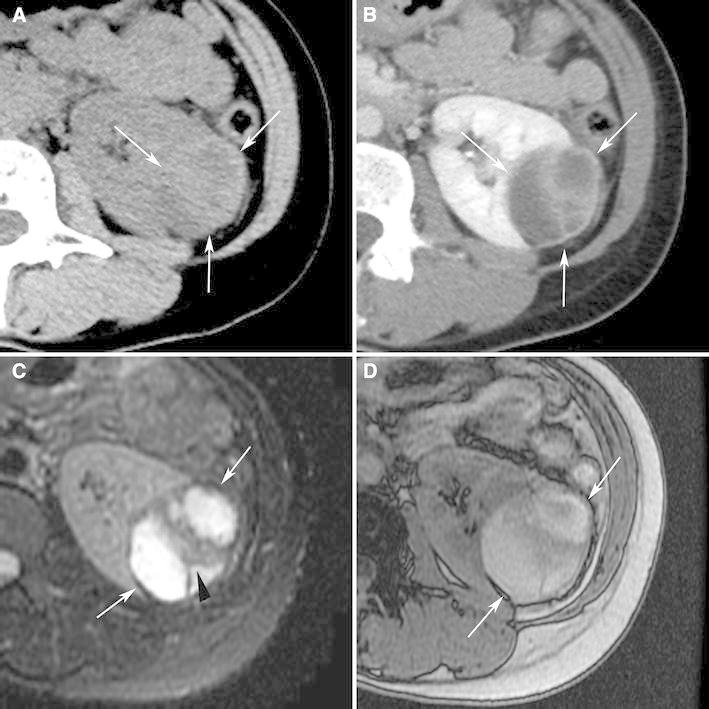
Epithelioid angiomyolipomae in a 40-year-old woman. Transverse, unenhanced CT (5-mm sections) (A) and enhanced CT (B) demonstrates a 5.0-cm multilocular cystic mass in the left kidney. Both the wall and septa (*arrows*) were hyperattenuating (48 HU) and enhanced. On transverse, T2-weighted MR image (fat-saturated fast spin-echo, TR:3800, TE: 89) (C), the wall (*arrows*) and septum (*arrowhead*) of the multilocular cystic mass appeared hypointense. On a transverse, T1-weighted MR image (fast spoiled gradient echo, TR: 150, TE:2.3) (D), the cystic component was hyperintense (*arrows*). The mass was considered a Bosniak Category 3 lesion and resected due to its appearance. The hyperintense cystic component corresponded to hemorrhage at pathology.
